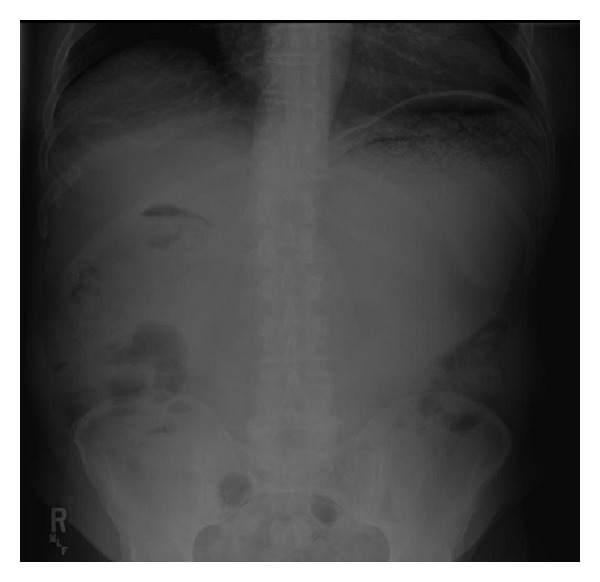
Abdominal X-ray showing dilated and partially fluid-filled stomach.
